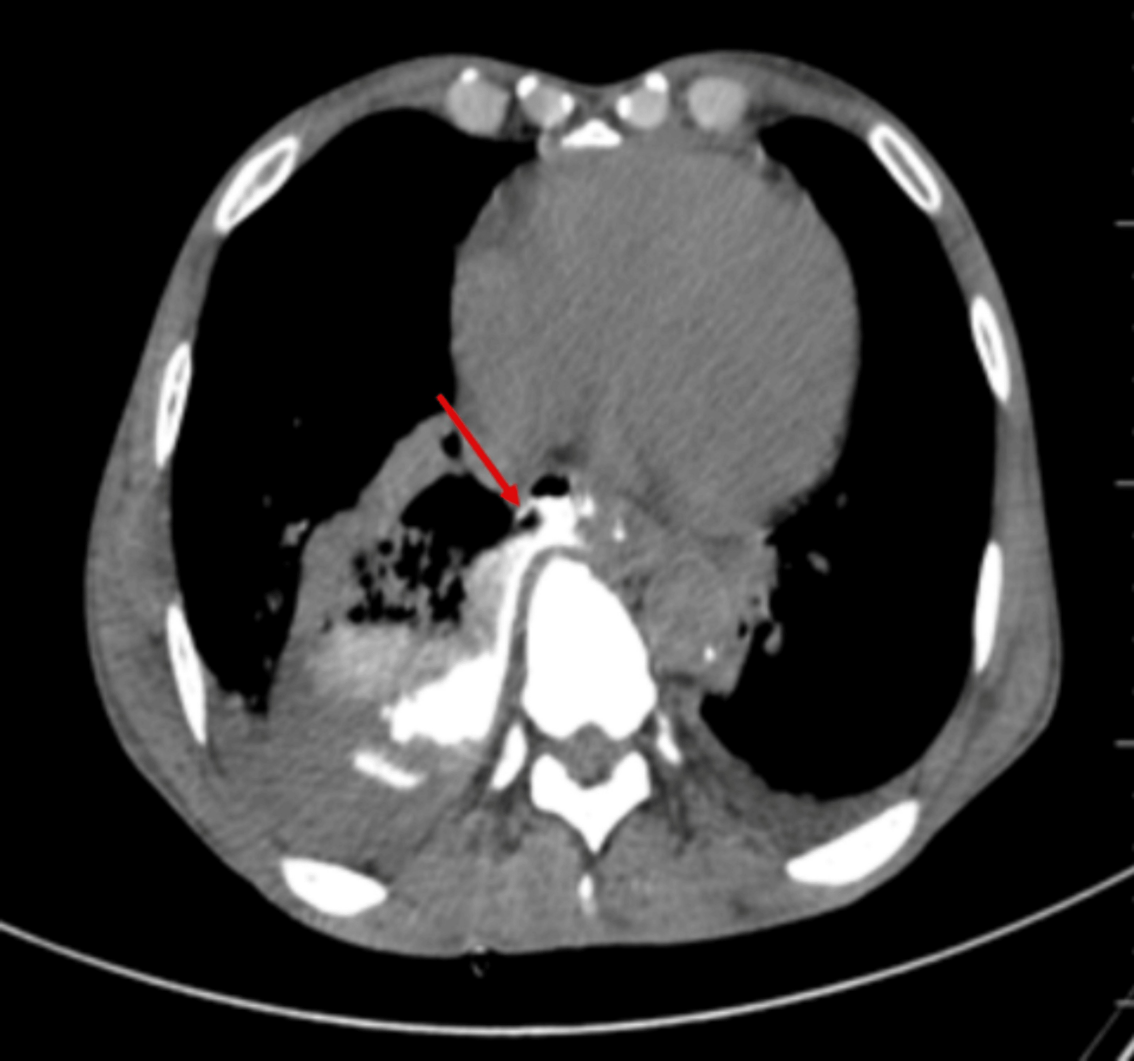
CT oral contrast phase - axial section The red arrow indicates the contrast tracking forming a fistulous communication into the lung parenchyma, resulting in the formation of a round, relatively well-defined, thick-walled peripheral enhancing collection in the right lower lobe parenchyma.
